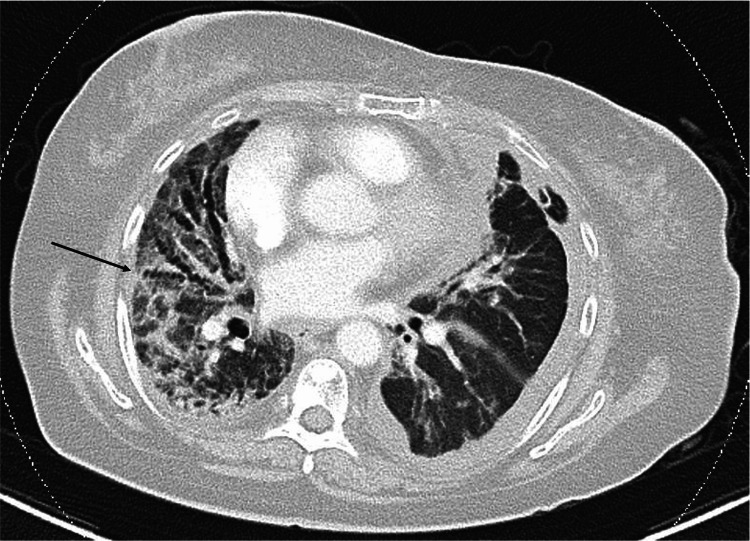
Computed tomography (CT) scan of the thorax done on D60 of admission, showing interval improvement of bilateral consolidation, with residual fibrosis (traction bronchiectasis and reticular infiltrates, as indicated by the black arrow)
